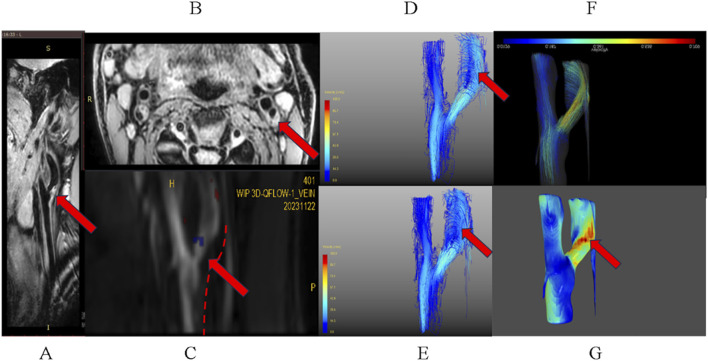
Plaque characterization with high-resolution MRI and 4D-flow hemodynamic analysis. (A) Coronal 3D T1 VISTA image showing a plaque at the origin of the left internal carotid artery (red arrow). (B) Axially reconstructed 3D-MERGE image of the same plaque. (C) Coronal view from GT-Flow software, showing the segmented lumen (dotted line) from 3D-Qflow data. The arrow indicates the plaque, visible as a filling defect. (D,E) Hemodynamic flow patterns represented by pathlines (D) and streamlines (E), reconstructed using GT-Flow. (F,G) Flow patterns streamlines, (F) and corresponding wall shear stress map WSS-Map, (G) reconstructed using the R language.
